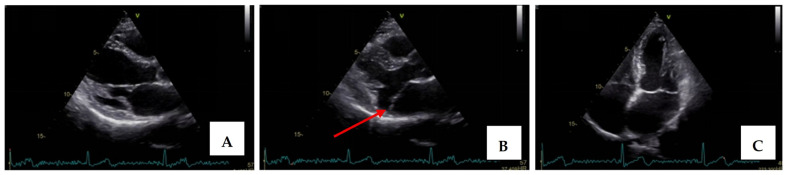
Mitral valve prolapse with MAD on transthoracic echocardiography (TTE). (A)—TTE parasternal long-axis view in end-diastole displaying excessive leaflet length and thickness, (B)—TTE parasternal long-axis view in end-systole displaying mitral annular disjunction-MAD of 10 mm length (red arrow), and (C)—apical 4-chamber view displaying a displacement of both leaflets > 2 mm above the plane of the annulus.
